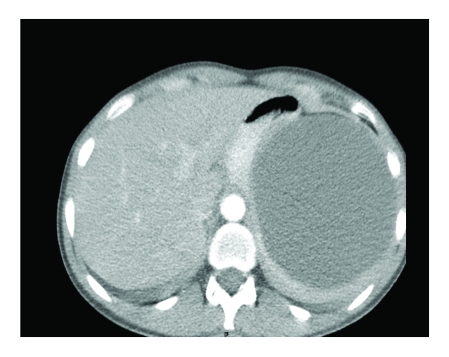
CT Image of the splenic cys
